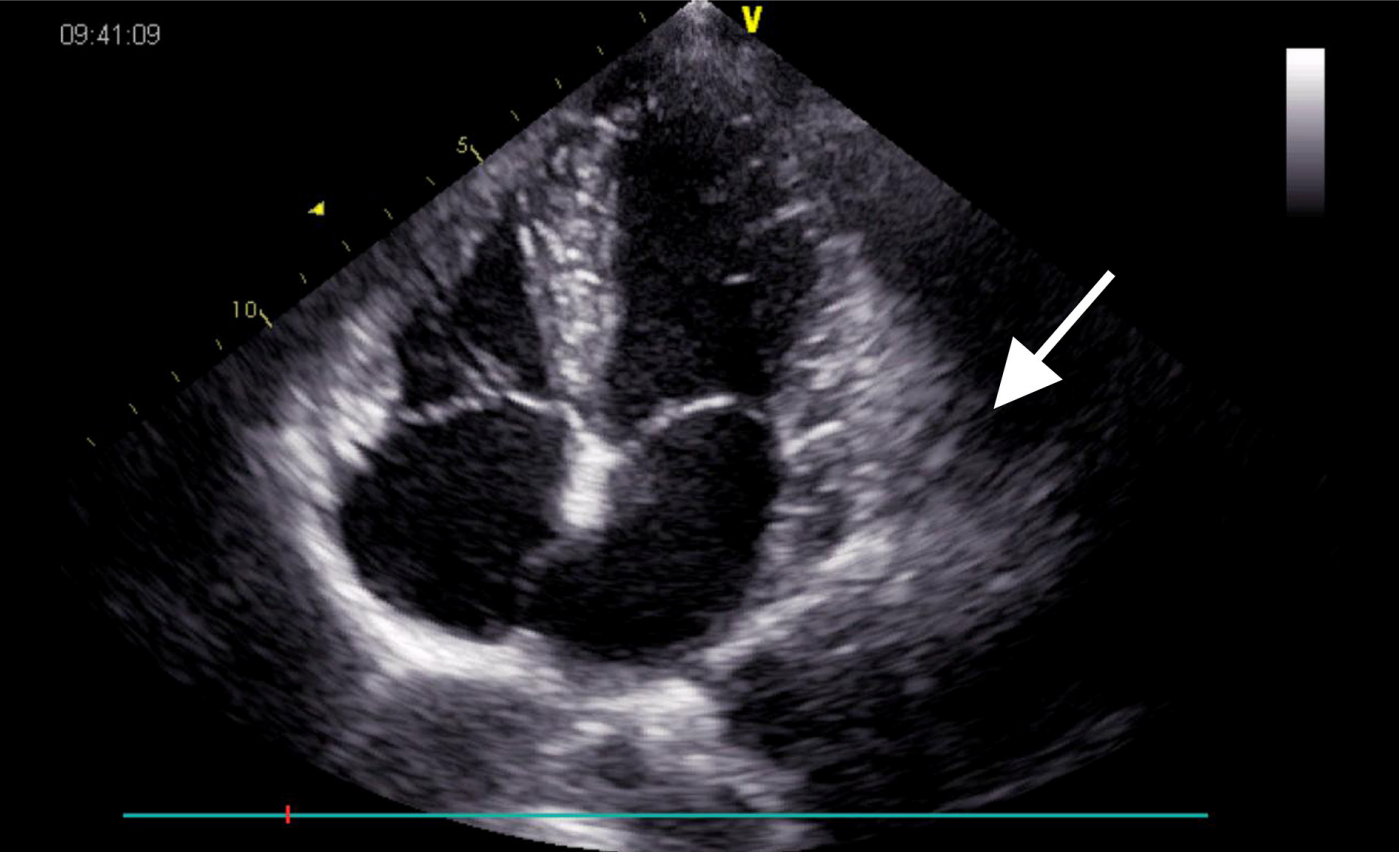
Echocardiogram pre-operatively showing a 32 × 66 mm heterogeneous mass (arrow) located in the left atrioventricular groove.
